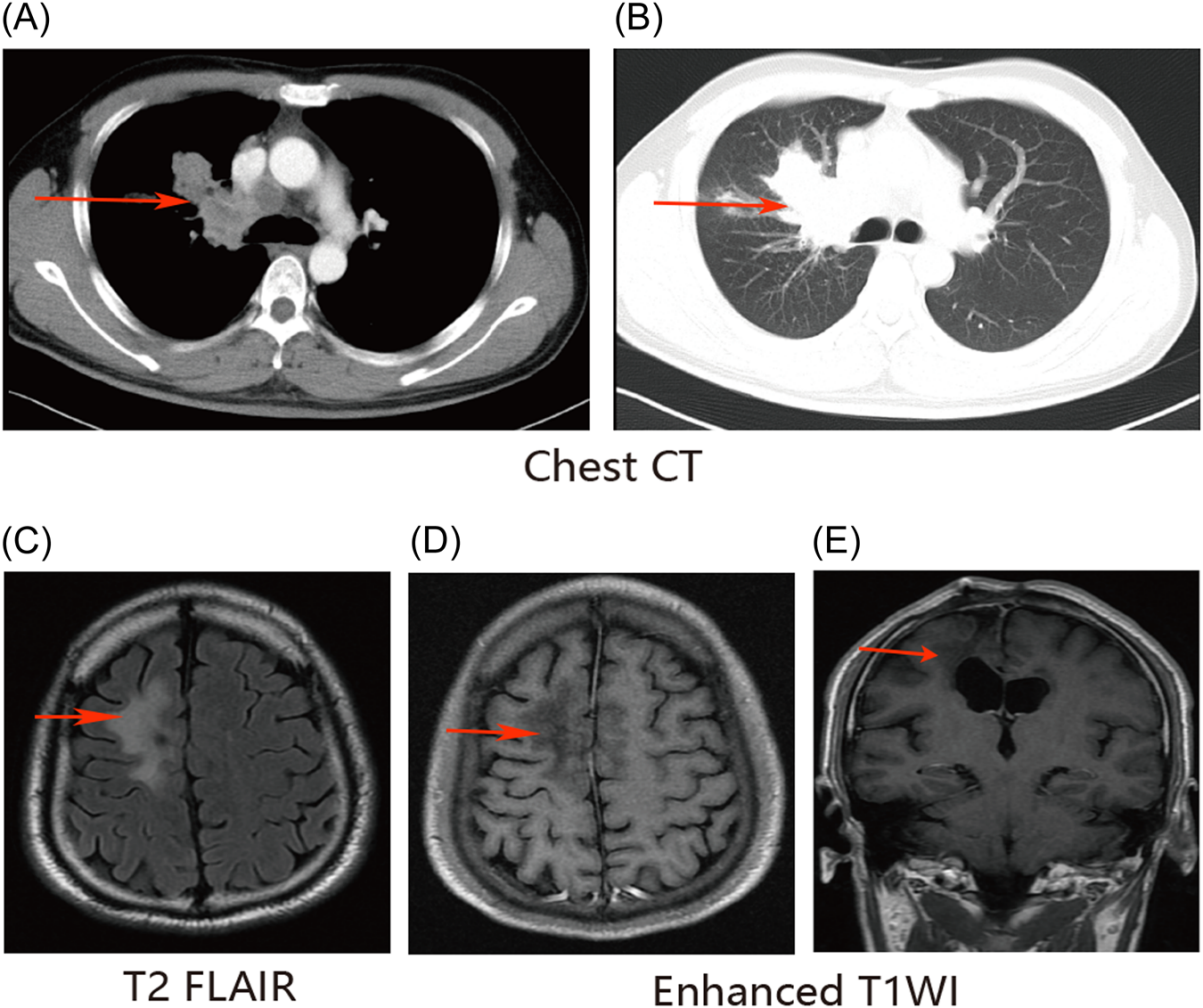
Results of computed tomography (CT) examination of lung and magnetic resonance imaging (MRI) examination of brain of patient when first seeing a doctor for hemoptysis (September 2016). CT examination of lung or MRI examination of brain, and arrows point to the lesion. [Color figure can be viewed at wileyonlinelibrary.com]
